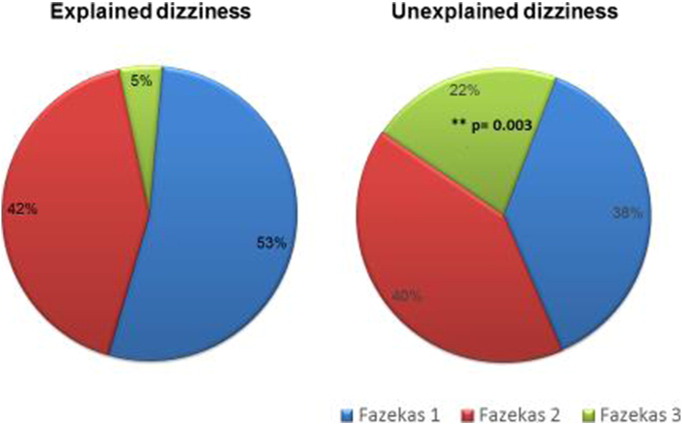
Severity of white matter disease on MRI (Fazekas scores), expressed as percentage of patients with “*explained*” and “*unexplained*” causes of dizziness.
